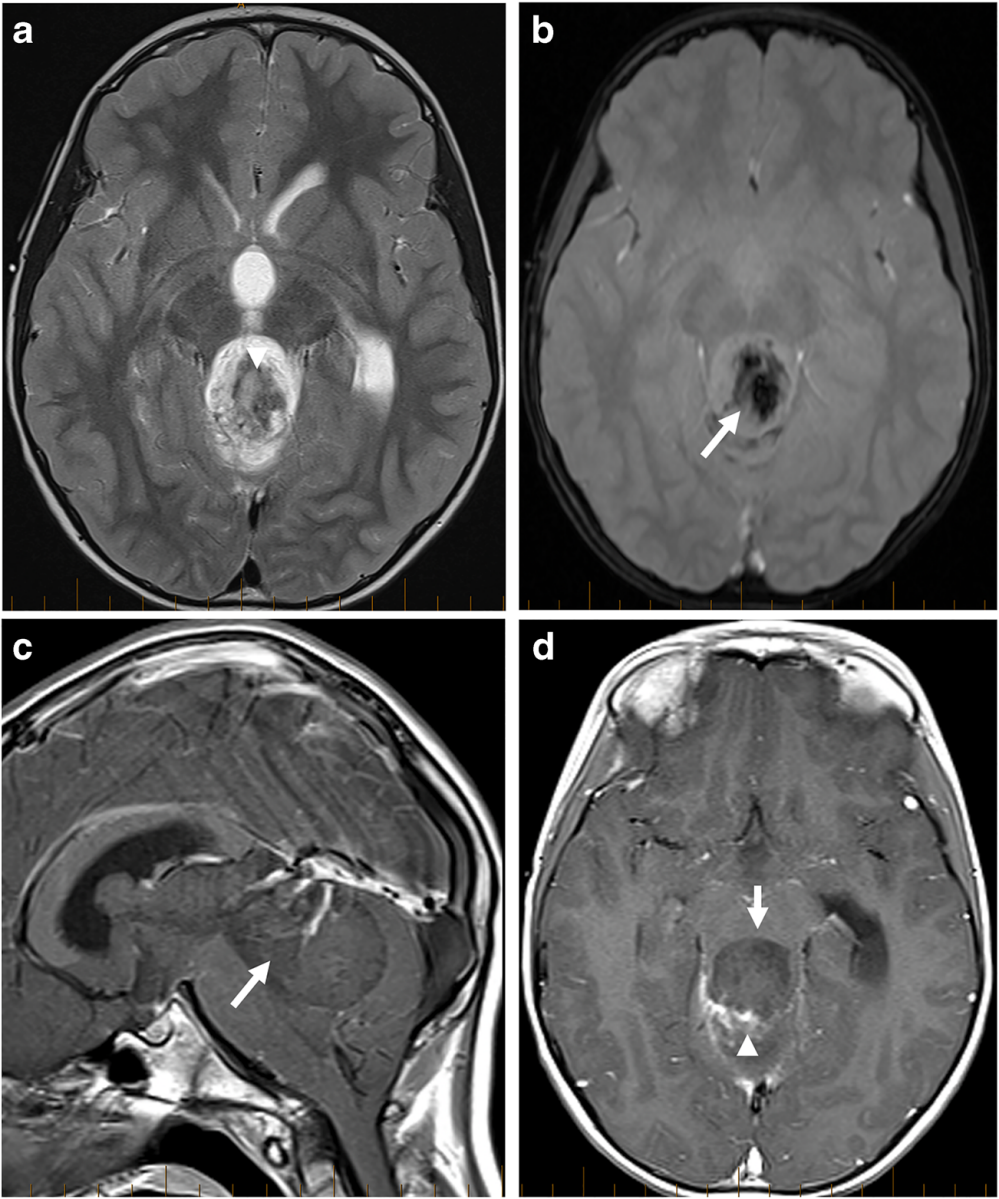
Typical imaging appearances of RGNT. The tumor involves the 4th ventricle and superior vermis and is heterogeneously hyperintense on T2-weighted sequence (a), mainly due to intratumoral hemorrhage that is better demonstrated on GRE images (arrow, b). Most of the tumor is nonenhancing (arrow, c, d), but areas of patchy enhancement (arrowhead, d) are seen on post-contrast T1-weighted sequences
